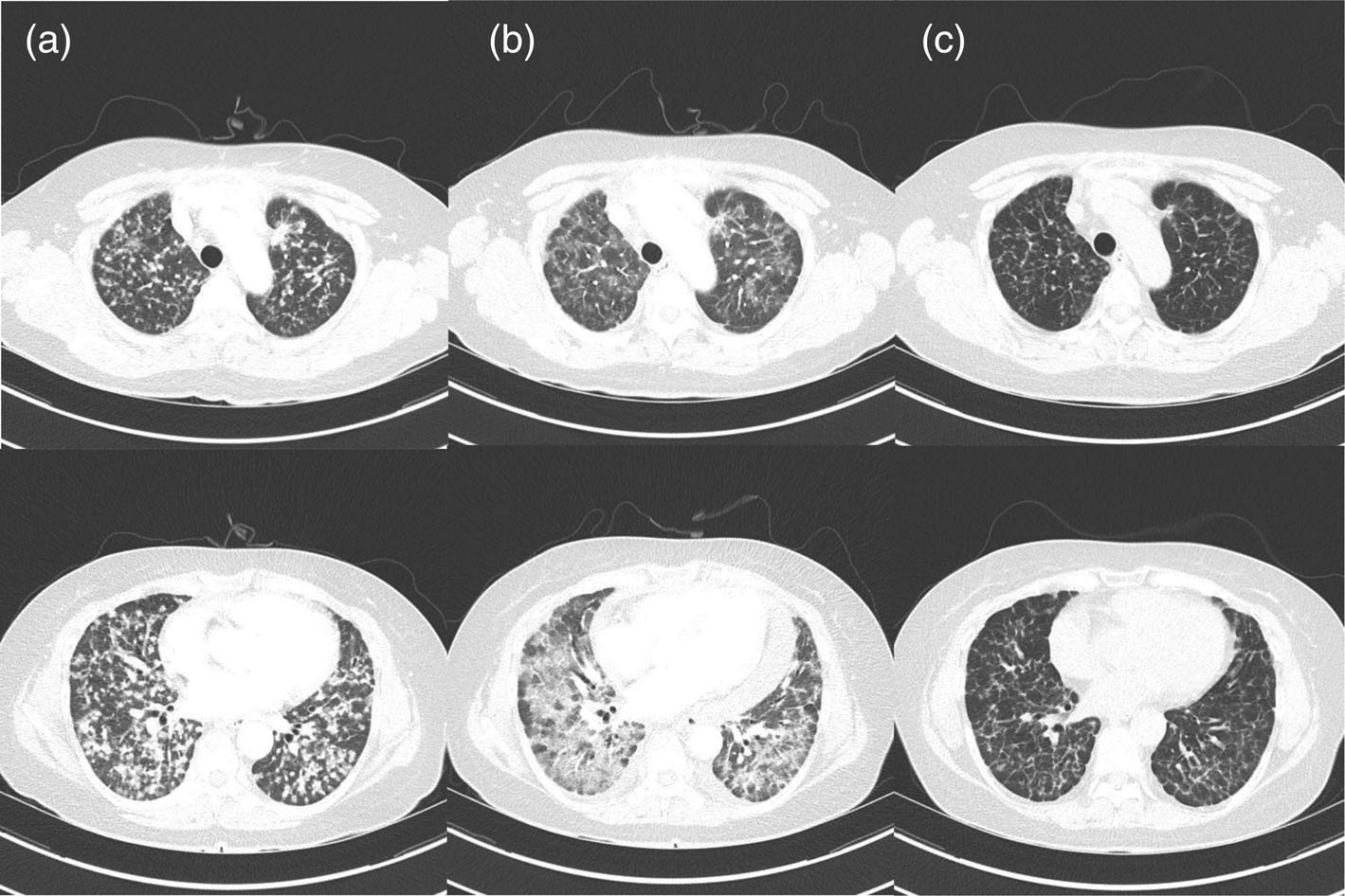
(a) Chest computed tomography (CT) scan showed multiple small well‐defined nodules in both lungs prior to administration of osimertinib. (b) Six days after administration of osimertinib, CT showed marked resolution of multiple tumor nodules in both lungs, but newly developed ill‐defined areas of patchy and extensive ground‐glass attenuation in the whole lung fields. (c) Four months after pneumonitis, CT showed partial remission of lung cancer with no evidence of recurrent pneumonitis.
